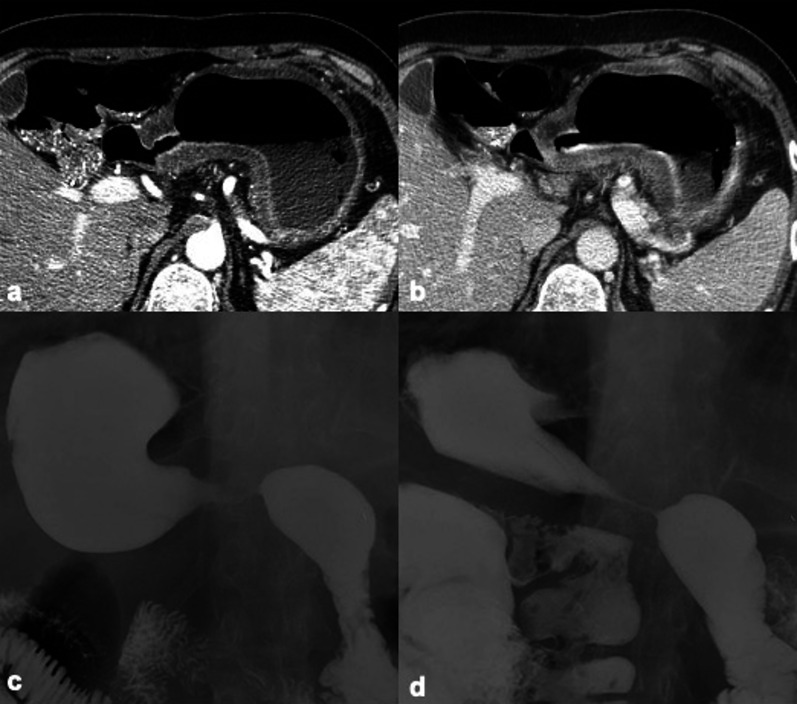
Gastritis due to checkpoint inhibitor therapy (Nivolumab). CT scans in arterial (a) and venous (b) phase showing linear enhancement of the gastric mucosa and diffuse, marked edematous thickening of the submucosal layer, more evident in the prepiloric area with concentric stenosis of the antral lumen. Moderate perigastric fat stranding is appreciable. The stenotic visceral segment is confirmed at barium fluoroscopy (c, d), however the passage of the contrast medium at the level of duodenum and small intestine is allowed
